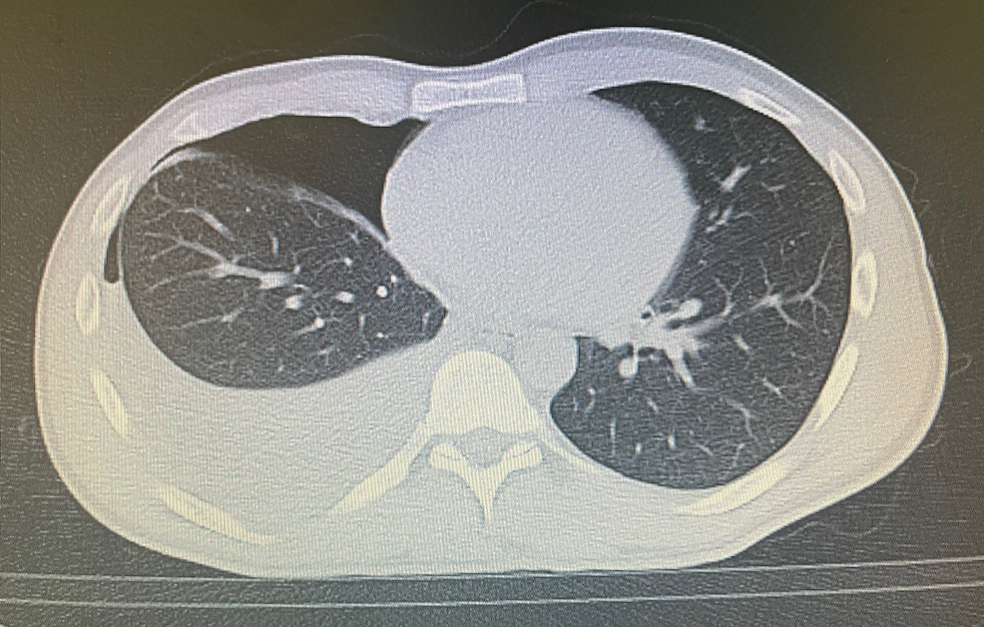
Chest computed tomography showing a large right-sided hydropneumothorax
